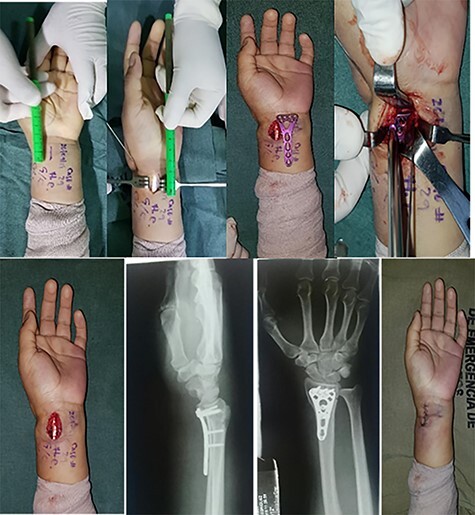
Longitudinal I shape incision. (a) Measurement of the incision. (b) The I incision. (c) The plate over the forearm. (d) Introducing the plate inside the forearm. (e) The forearm with the plate introduced. (f) Lateral X-ray of the wrist. (g) Anteroposterior X-ray of the wrist. (h) The incision already sutured.
